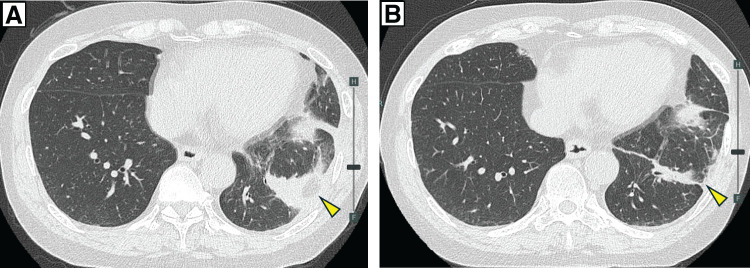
Follow-up CT at 1 month (A) and 4 months (B) postoperatively shows no residual foreign body, abscess, or granulomatous lesion. The surrounding consolidation had nearly resolved. The sutured pericardial fat tissue (arrowheads) appeared stable at 1 month but had decreased in size by 4 months.
